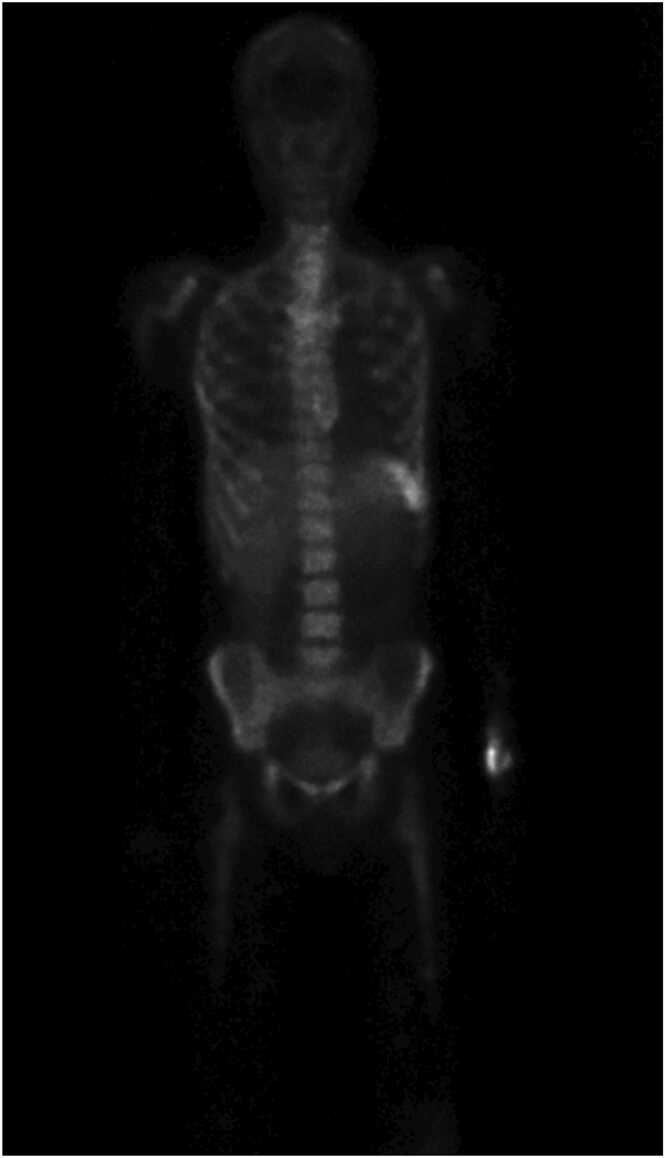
Anti-granulocyte scintigraphy showed no evidence of disseminated infection following surgeries and antibiotic treatment.
